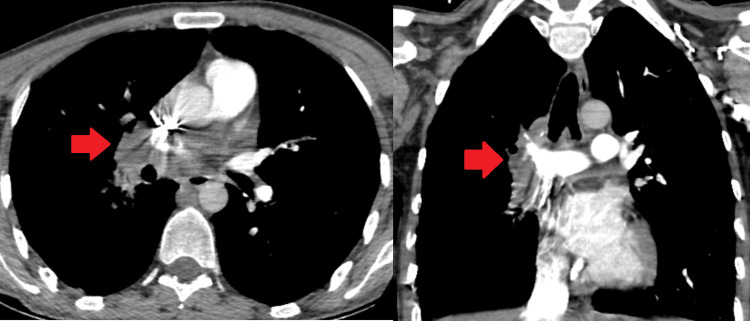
Follow-up CT chest After six months of treatment, the size of the aneurysmatic lesions increased, and hence the duration of treatment was extended CT: computed tomography
